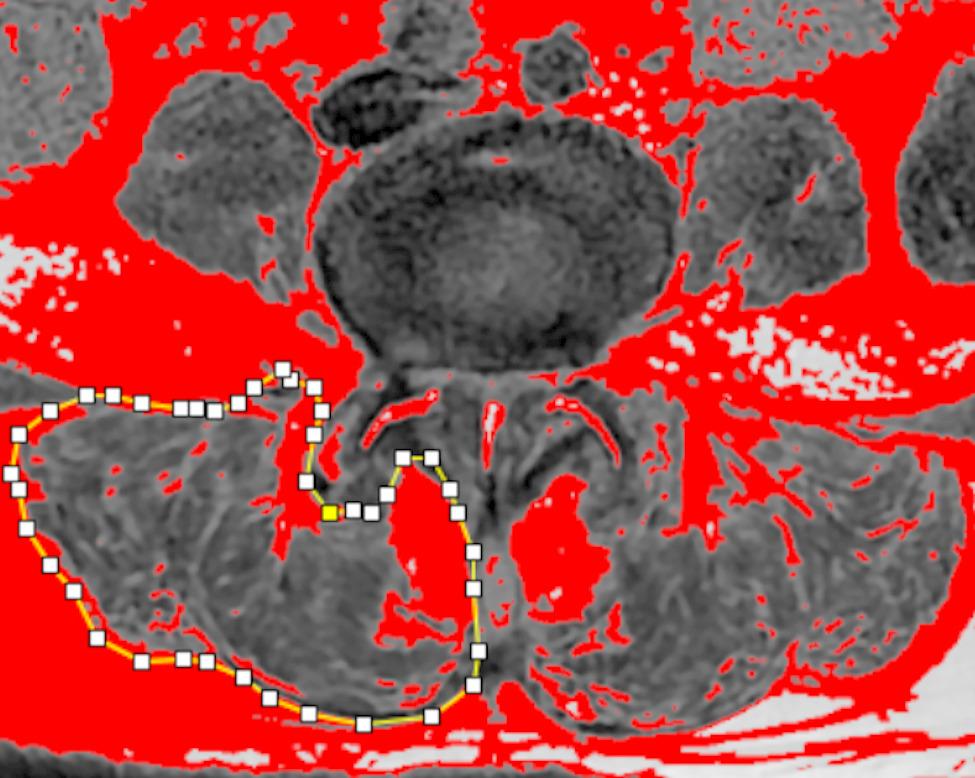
The image was processed using Image J software, with solid and dotted lines outlining the paravertebral muscle region. Fat infiltration (FI) of the paravertebral muscles was measured by applying the thresholding technique in Image J to T2-weighted MRI cross-sectional images of the lumbar spine, where red areas indicated fat infiltration. FI was then quantified for each paravertebral muscle region using the software tools
